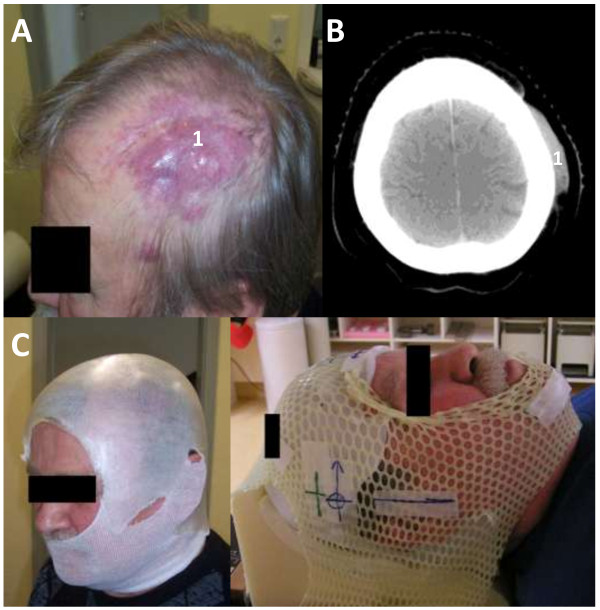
Clinical presentation and treatment setup in patient 2. (A) Clinical presentation of patient 2 with cutaneous manifestation of B-NHL (1) indicates tumor lesion (B) pre-treatment transversal CT scan with scalp tumor (treatment planning CT). (C) treatment setup (left) head cover and custom-made individual bolus on top (right) immobilization mask with bolus underneath.
